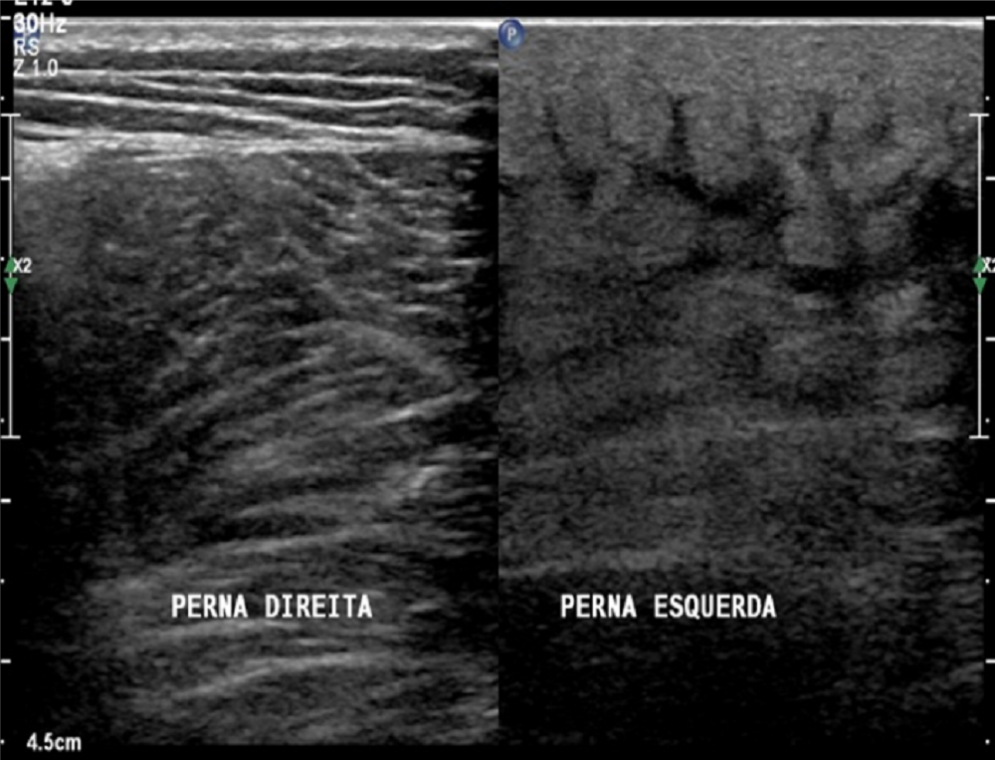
High-frequency ultrasound. Left side of the image: normal right lower limb; right side of the image: left lower limb with lymphedema and disarrangement of skin structures
